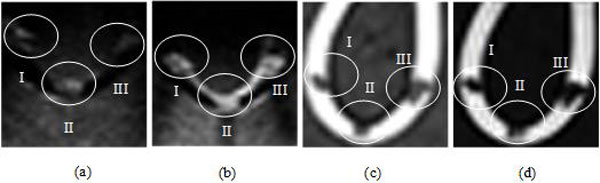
Enhancement imaging based on MMBs. (a) US image without MMBs. (b) US image with MMBs. (c) MRI without MMBs. (d) MRI with MMBs.
